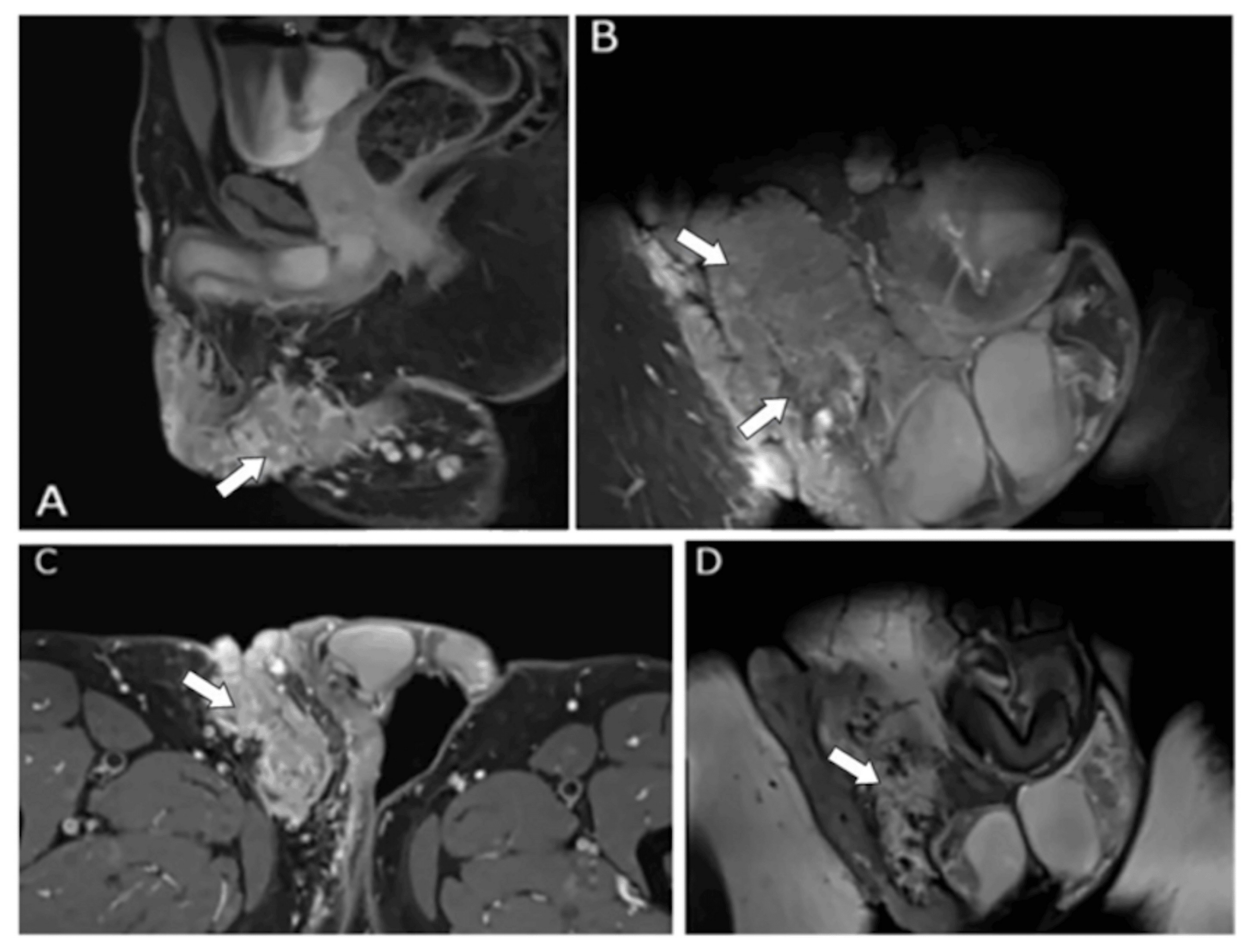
MRI scans. (A) Sagittal image. (B) Coronal image. (C) Traverse image. (D) Coronal image. Solid tissue mass anteriorly over the skin in front of the penis, along the right proximal thigh, reaching the inferomedial surface of the perineum, the right inguinal-genital region, and the skin over the right hemiscrotum. Pathologically enlarged lymph nodes are noted in the pelvic floor.
